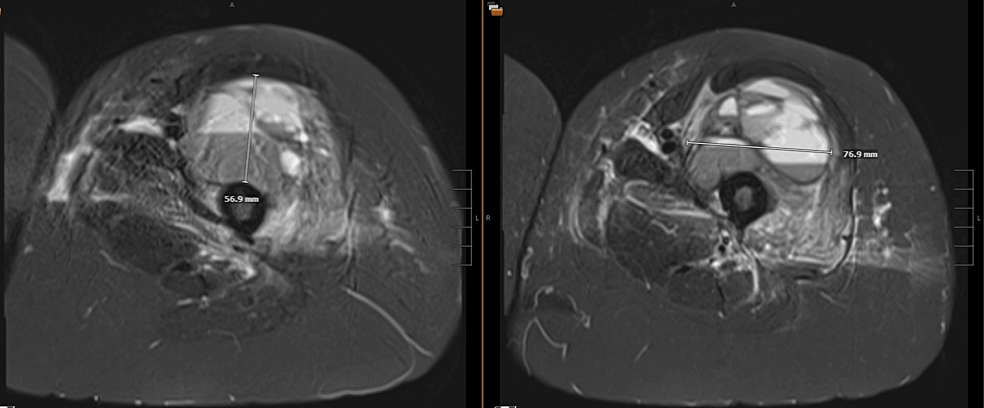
Left femur MRI - Multilobulated multiseptated lesion identified within the anterior compartment musculature centered in the vastus intermedius. The mass measured 76.9 mm x 56.9 mm.
